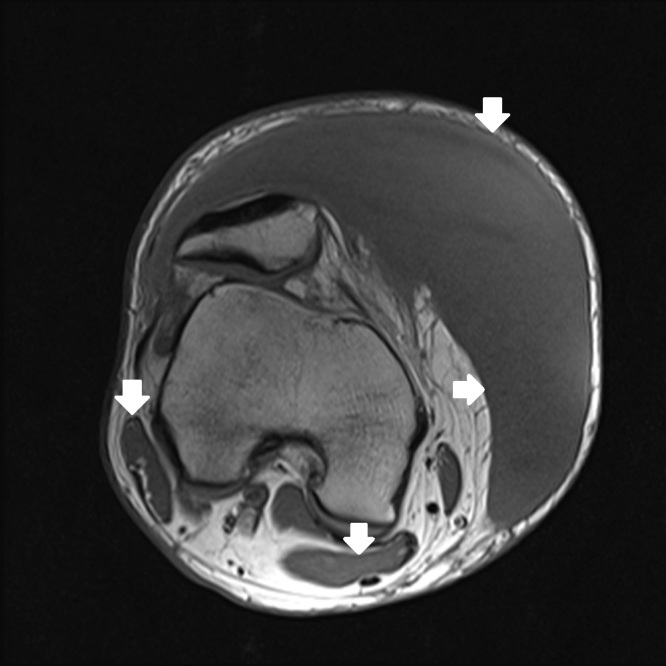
Axial T1-weighted magnetic resonance imaging shows the lesion is isointense to muscle.
